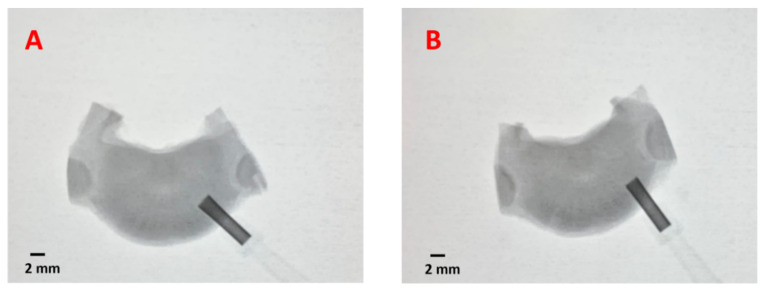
X-ray representative images of the biopsy punch-induced annulus fibrosus defect creation in (A) untreated and (B) PNCOL-treated IVDs. (n = 4).
